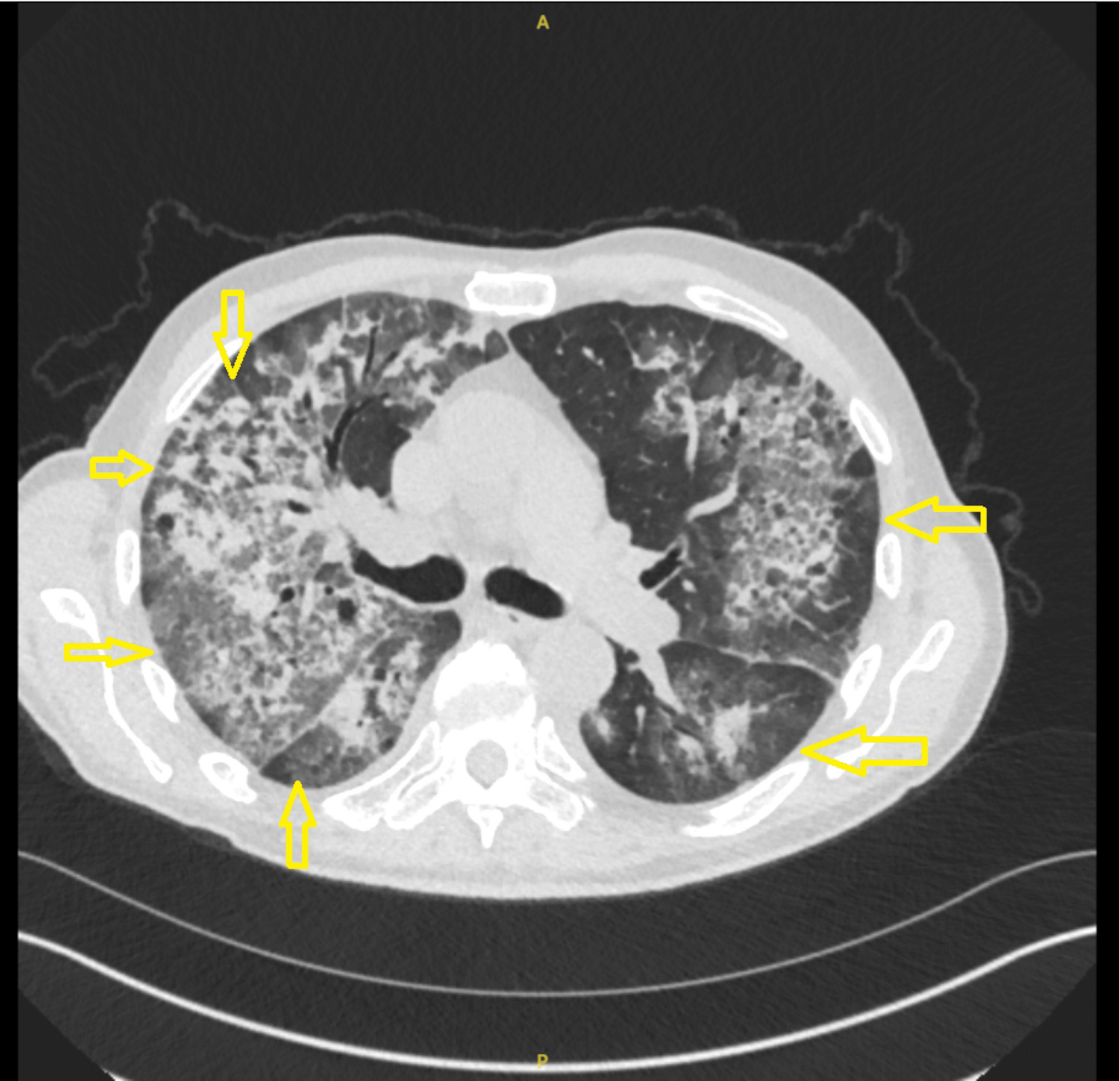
CT chest showing bilateral widespread pulmonary consolidations, more pronounced on the right side; findings suggest the need to rule out pulmonary edema, hemorrhage, viral pneumonia, or Pneumocystis pneumonia.
